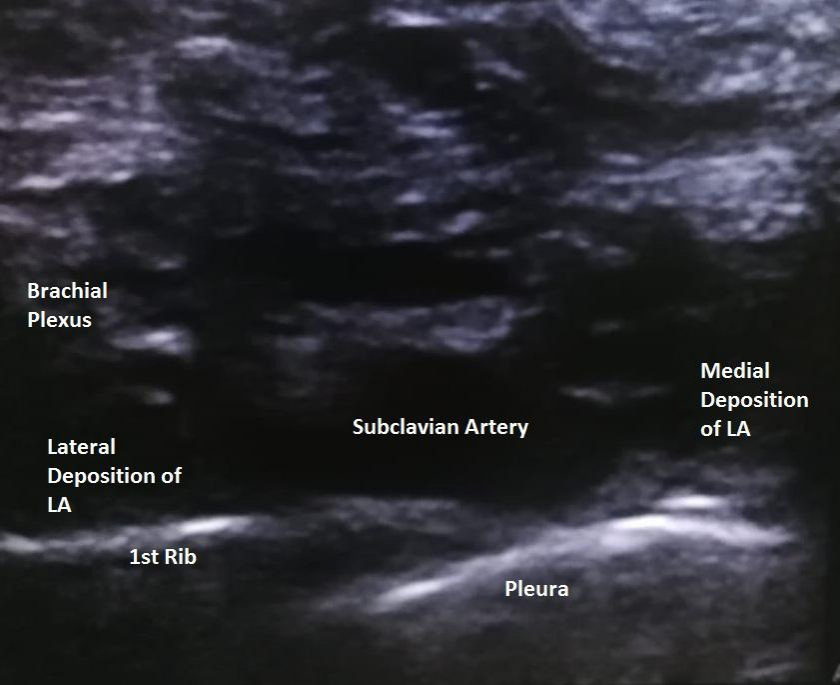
Ultrasound image after block.
